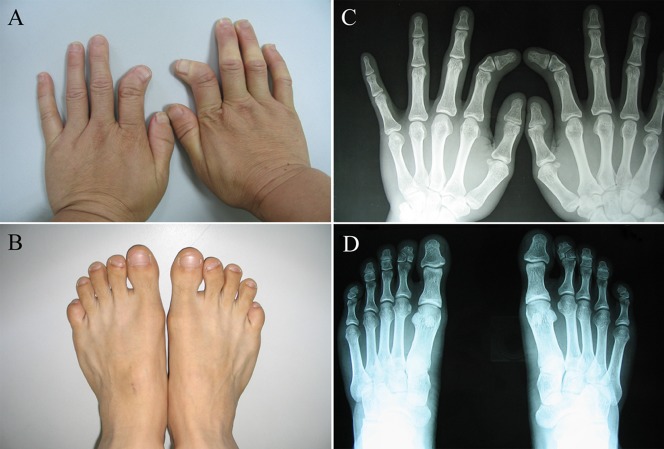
Phenotype of BDA2. A and B: Short and medially deviated index finger (above) and second toe (below). C and D: Radiograph of the triangular-shaped middle phalanx of the index finger (above) and the second toe (below).
